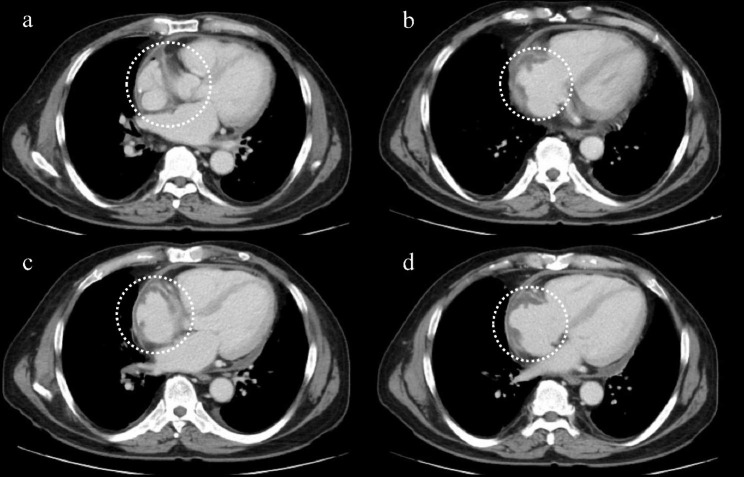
Contrast-enhanced computed tomography on admission The images are horizontal sections from a, b, c, and d, arranged from cranial to caudal order. Low-density area along the right atrial wall
